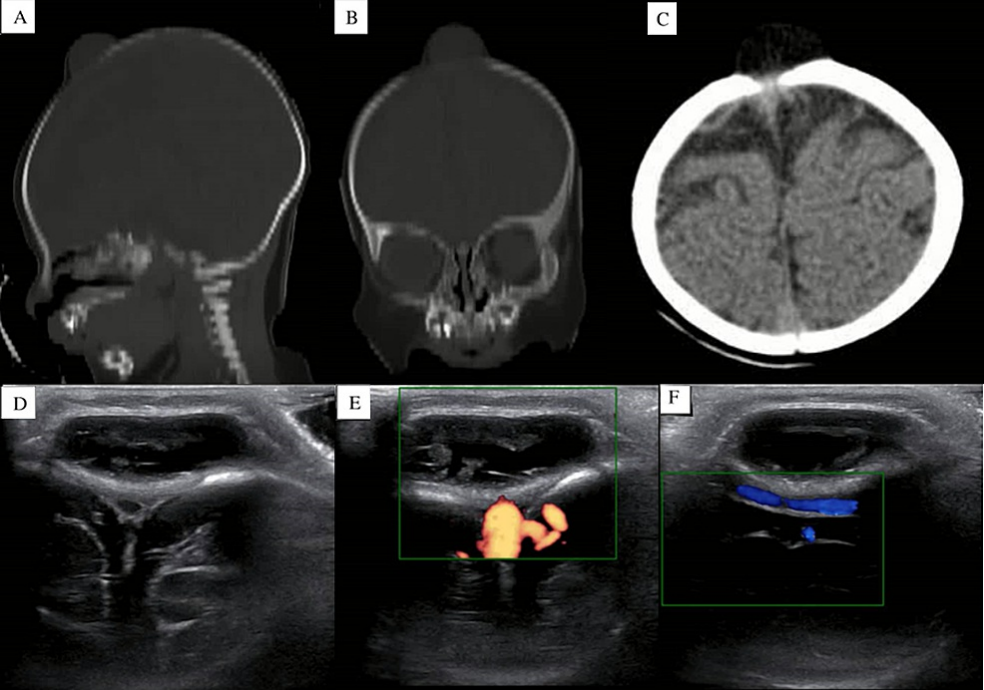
CT and Doppler US A) Head CT from a sagittal view. B) Head CT from a coronal view. C) Head CT from an axial view. In all of them, we can appreciate an extracranial mass, consistent with an anterior fontanelle dermoid cyst. D) Ultrasound from a coronal view. E) Doppler Ultrasound from a coronal view. F) Doppler Ultrasound from a longitudinal view. In neither of them, we see blood flow inside the cyst.
